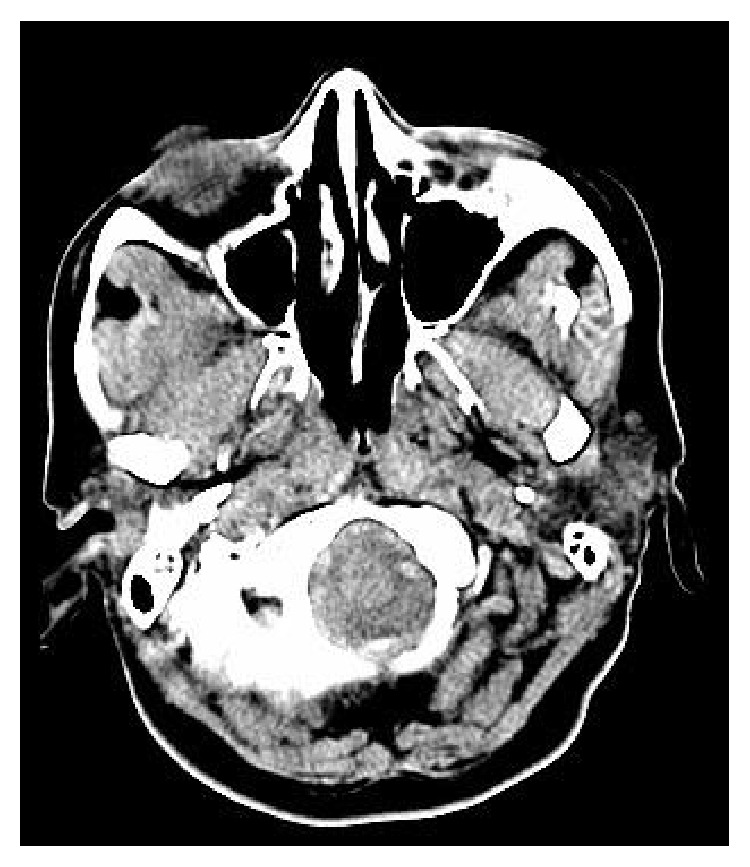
Cranial CT images of patient 3.
